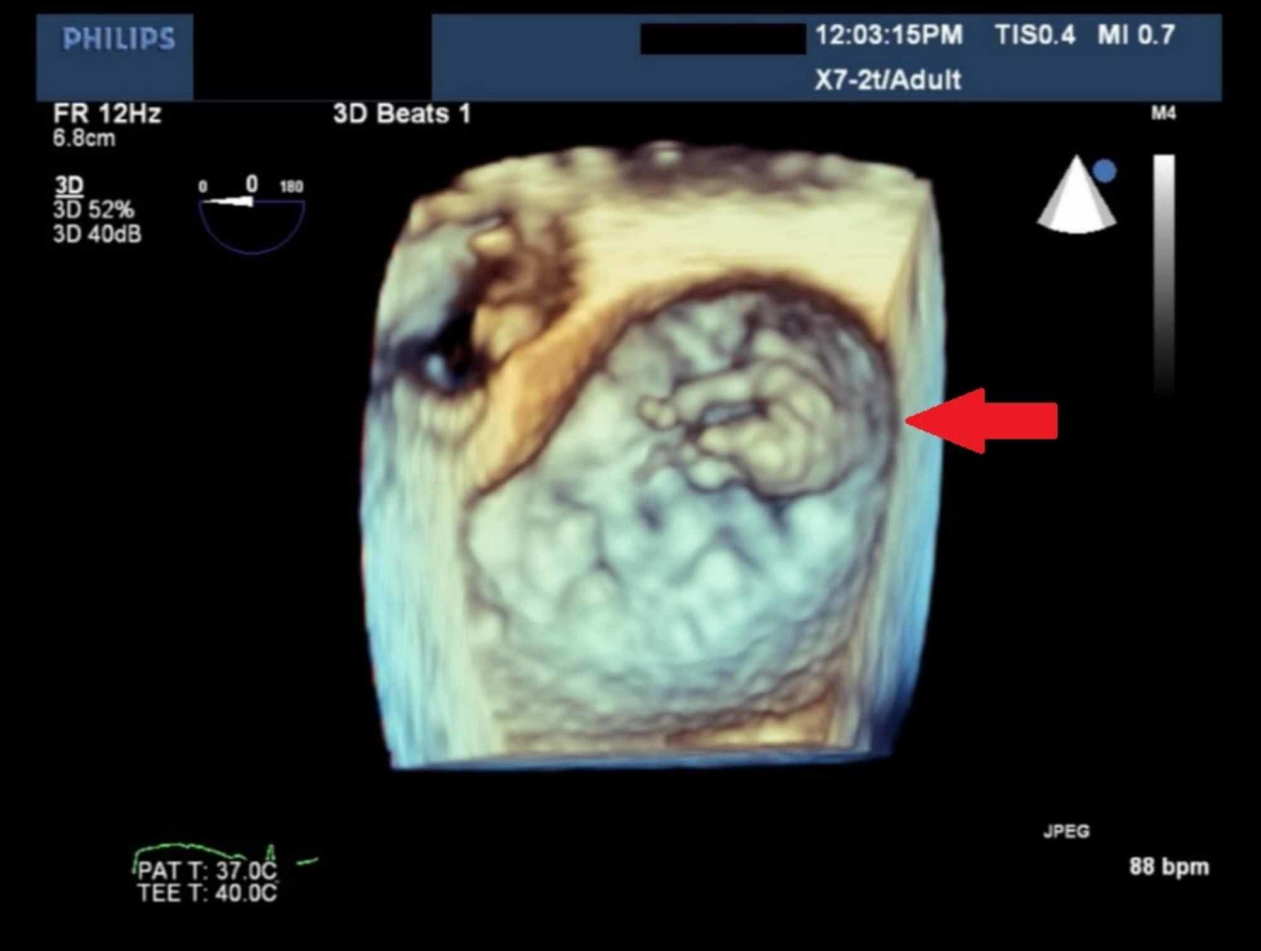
Transesophageal echocardiogram with 3D image acquisition Evidence of a flailed P3 scallop of the mitral valve leaflet. There is severe eccentric, anteriorly directed mitral regurgitation.
